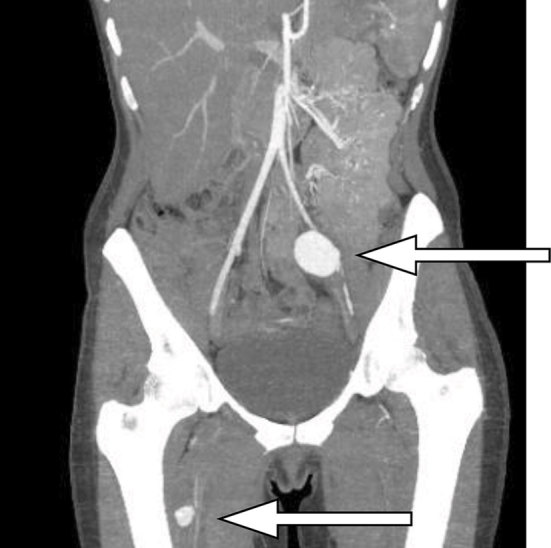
Arterial phase contrast CT (maximum intensity projection) showing left common iliac and right profunda femoris artery aneurysms.
